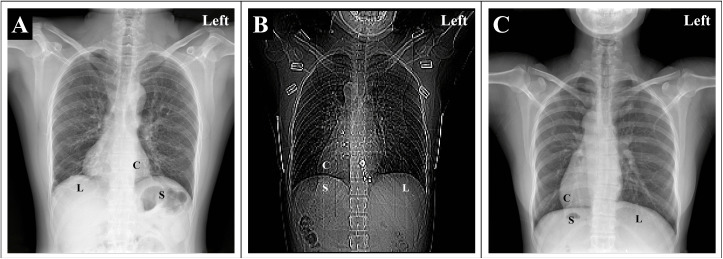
Radiology imaging of the three patients. (A) Chest X-ray of the patient 1 diagnosed with situs solitus revealed dextroposition, left stomach bubble, and right-sided liver. (B) Computed tomography of the patient 2 diagnosed with situs inversus revealed right cardiac apex, right stomach bubble, and left-sided liver. (C) Chest X-ray of the patient 3 diagnosed with situs inversus revealed right cardiac apex, right stomach bubble, and left-sided liver. C, cardiac apex; S, stomach bubble; L, liver.
